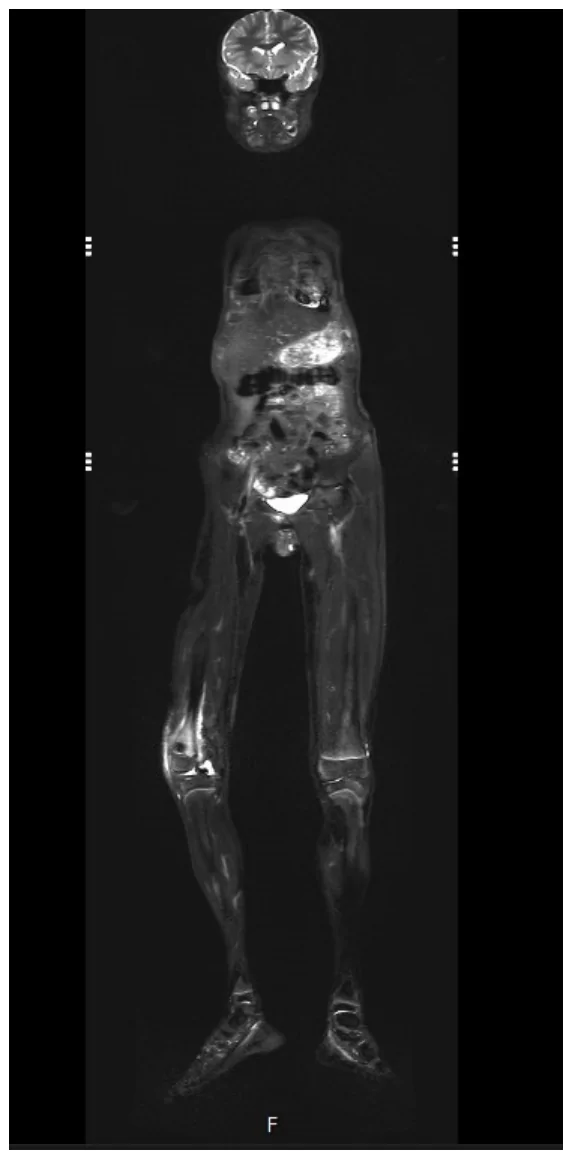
Whole-body MRI using DWIBS in a patient with CNO without bone destruction. Foci of bone marrow edema in the distal epiphysis of the right tibia and in the left femur.
